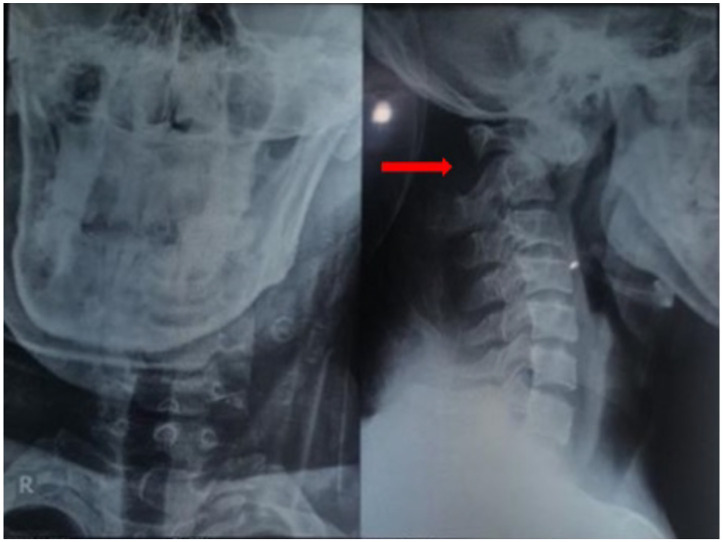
A/P Lateral X-rays of the cervical spine showed fracture at the axis (C2), specifically at the odontoid process, categorized as type II, based on Anderson and D'Alonzo classification (arrows).
